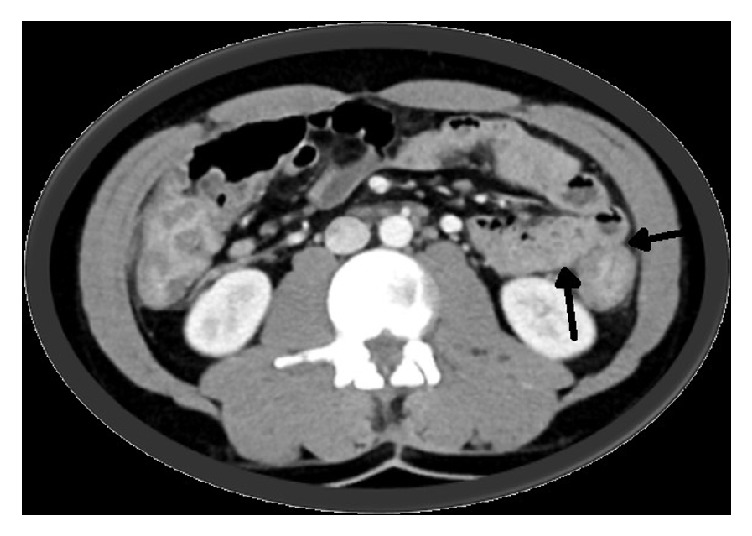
Transverse abdomen CT. Diffuse abnormal colonic thickening consistent with pancolitis (arrows). The small bowel is normal.
